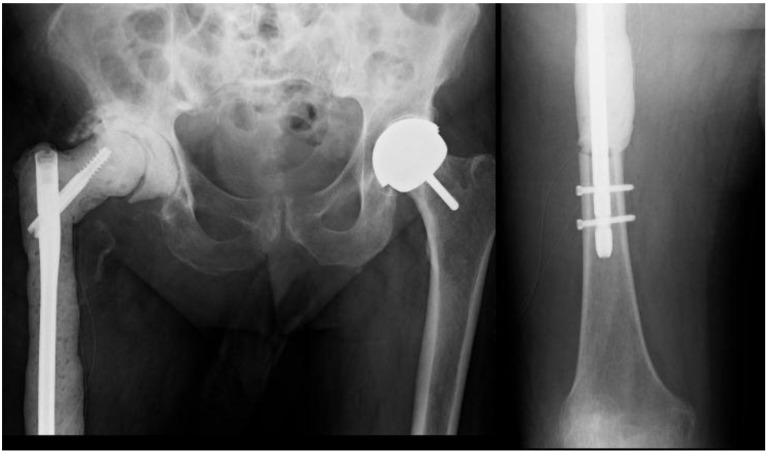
Postoperative X-rays showing the temporary antibiotic spacer construct. Note the antibiotic cement does not surround the nail within the bone, as opposed to a pre-constructed antibiotic nail.
